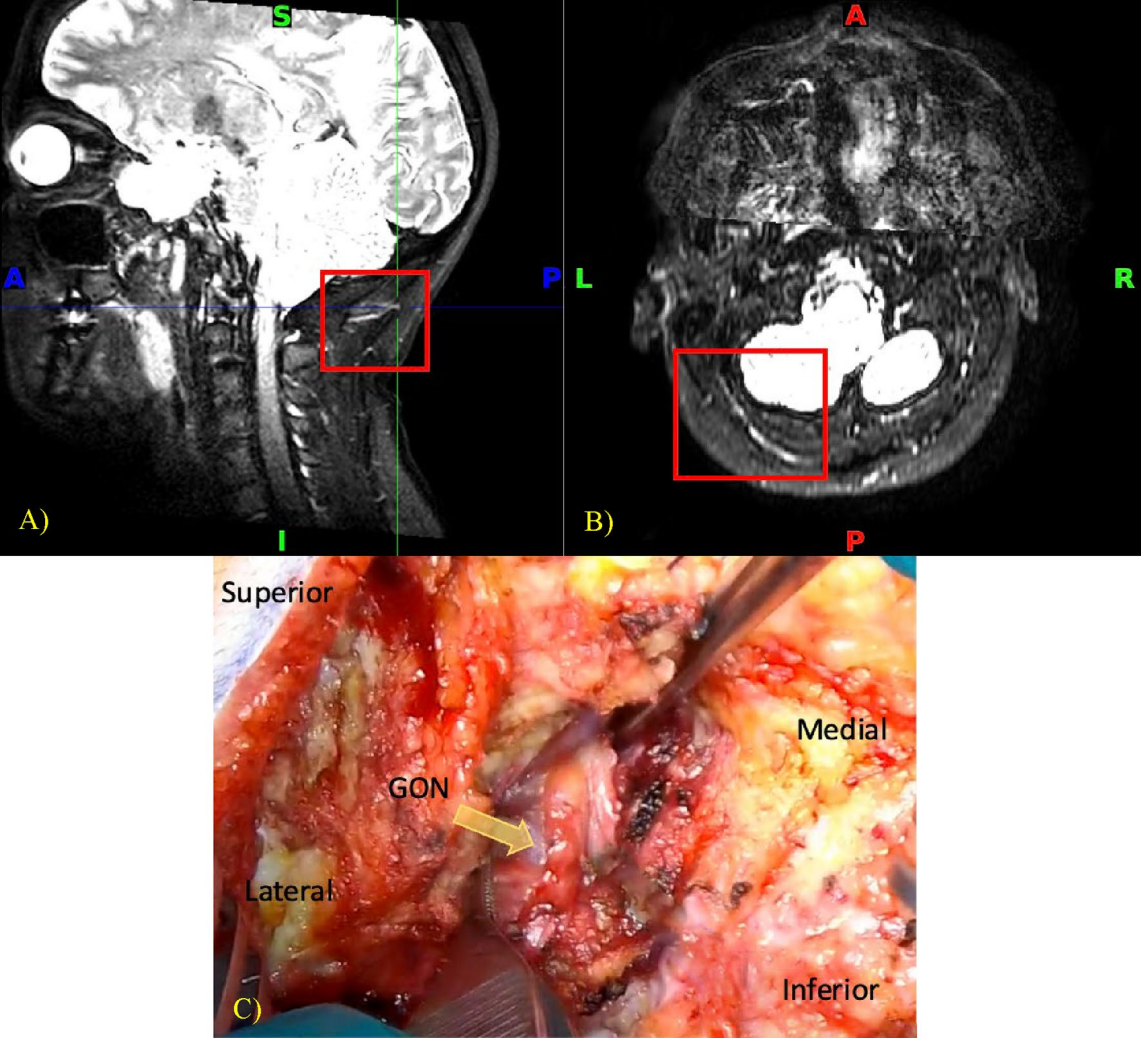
(A) Sagittal and (B) Transverse NerveView MRI tracking the left GON with high intensity signal as it courses through the trapezius fascia (red box). (C) Intraoperative thickened and yellowish GON at the level of a thick trapezius fascia (yellow arrow).
